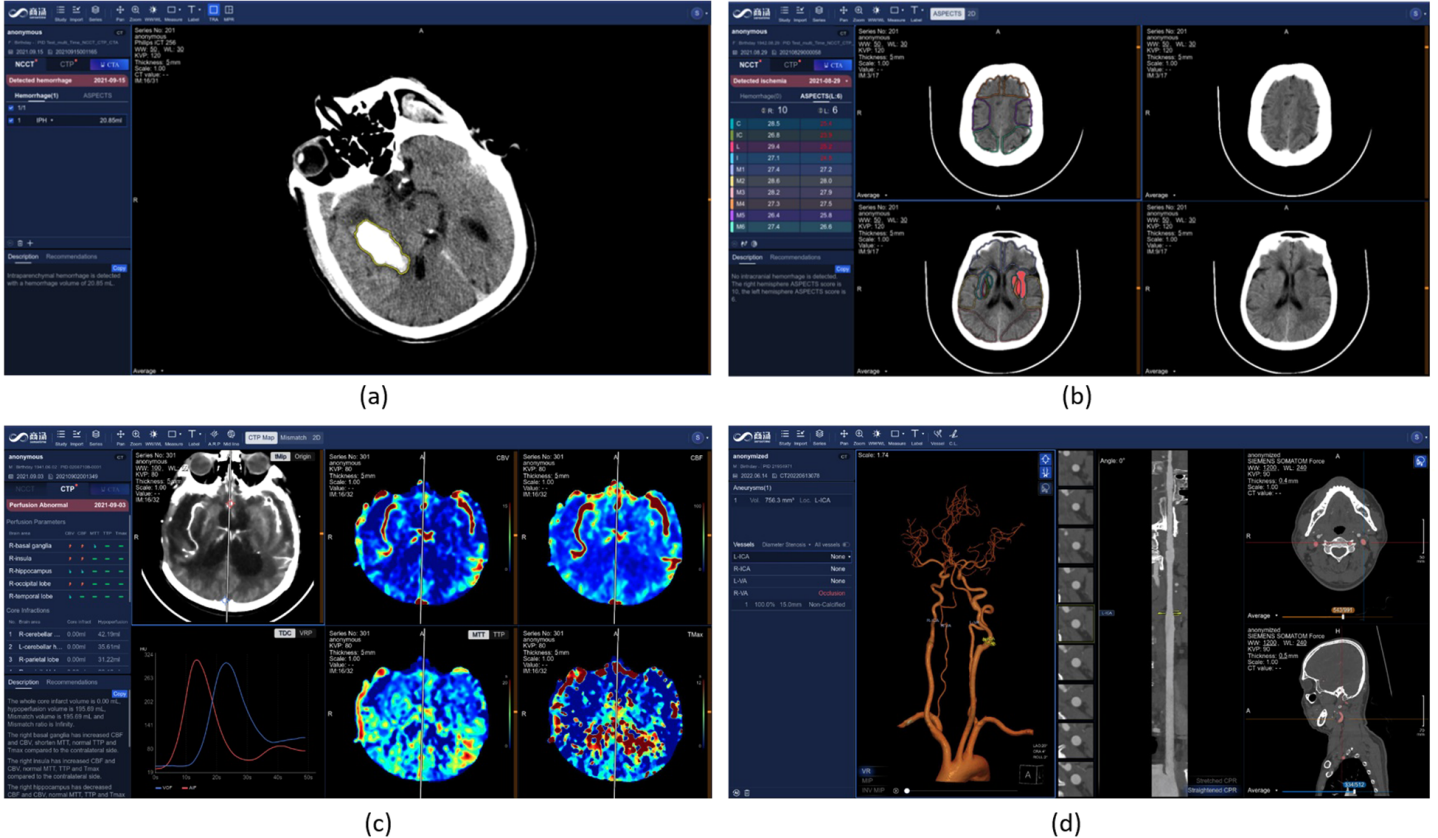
Stroke diagnosis in SenseCare. (a) Hemorrhage diagnosis. (b) ASPECT score measurement for ischemic stroke. (c) CT perfusion analysis. (d) Head and neck CTA analysis.
